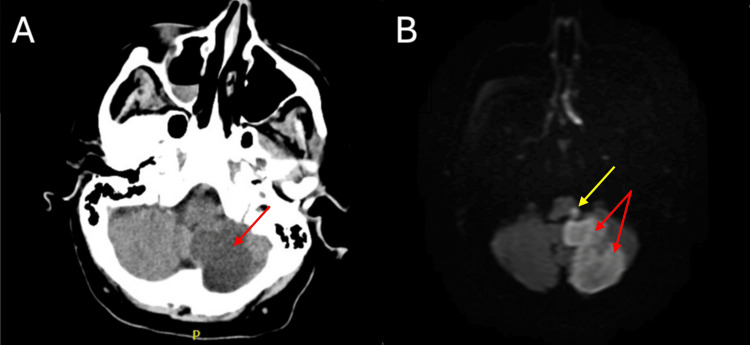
Repeat CT of the head (A, B) showing the previous infarct of the left cerebellum (red arrows) and left medulla (yellow arrow) without evidence of hemorrhagic transformation CT: computerized tomography
